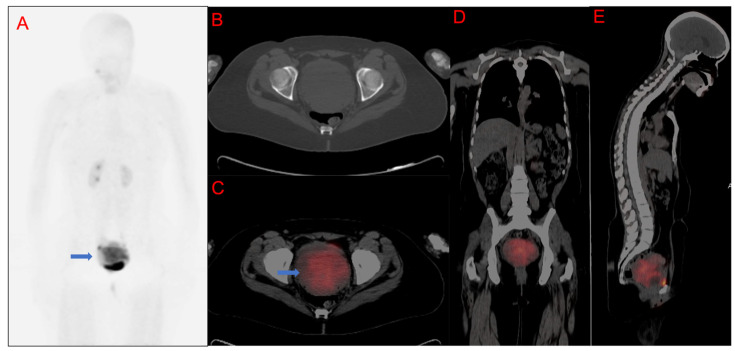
A 36-year-old female with moderately differentiated squamous cell carcinoma of the cervix. [^68^Ga]Ga-FAPI-46 maximum intensity projection images (MIP), (A). CT only axial slice through the pelvis, (B). Fused PET/CT axial images through the pelvis, (C). Whole-body fused PET/CT coronal images, (D). Whole-body fused PET/CT sagittal images, (E). Demonstrating a huge primary tumor (blue arrows) with intense [^68^Ga]Ga-FAPI-46 uptake. SUVmax = 24.41, FAPI-TV = 262.50 cm^3^.
